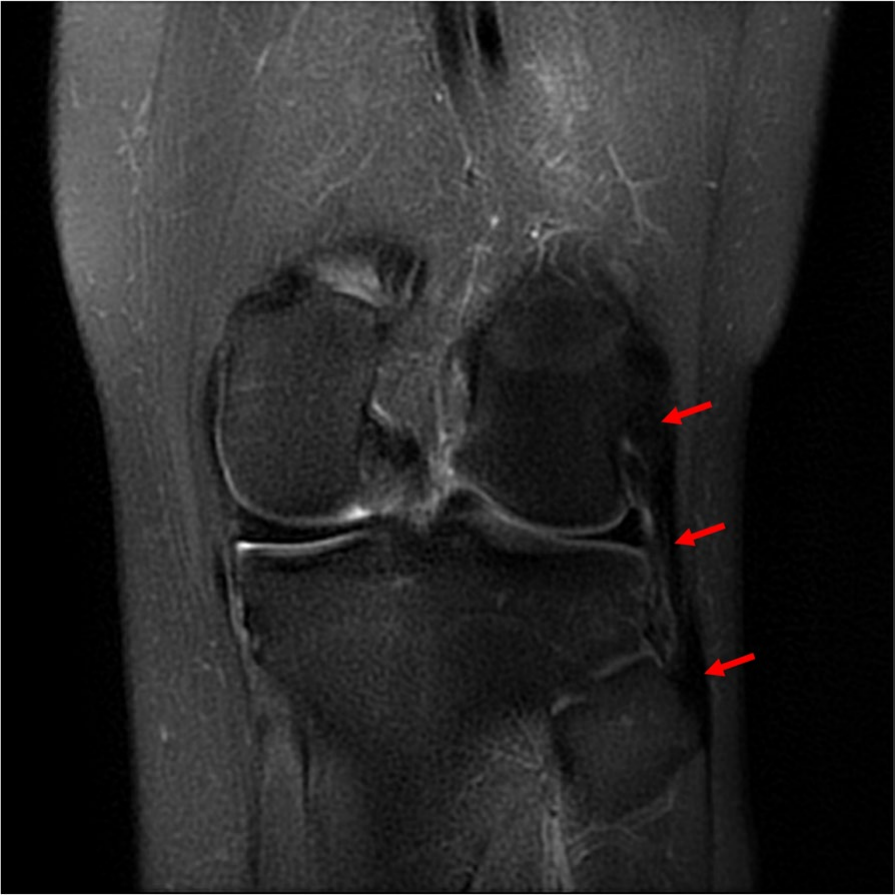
Positive coronal LCL sign (red arrows) in magnetic resonance imaging
